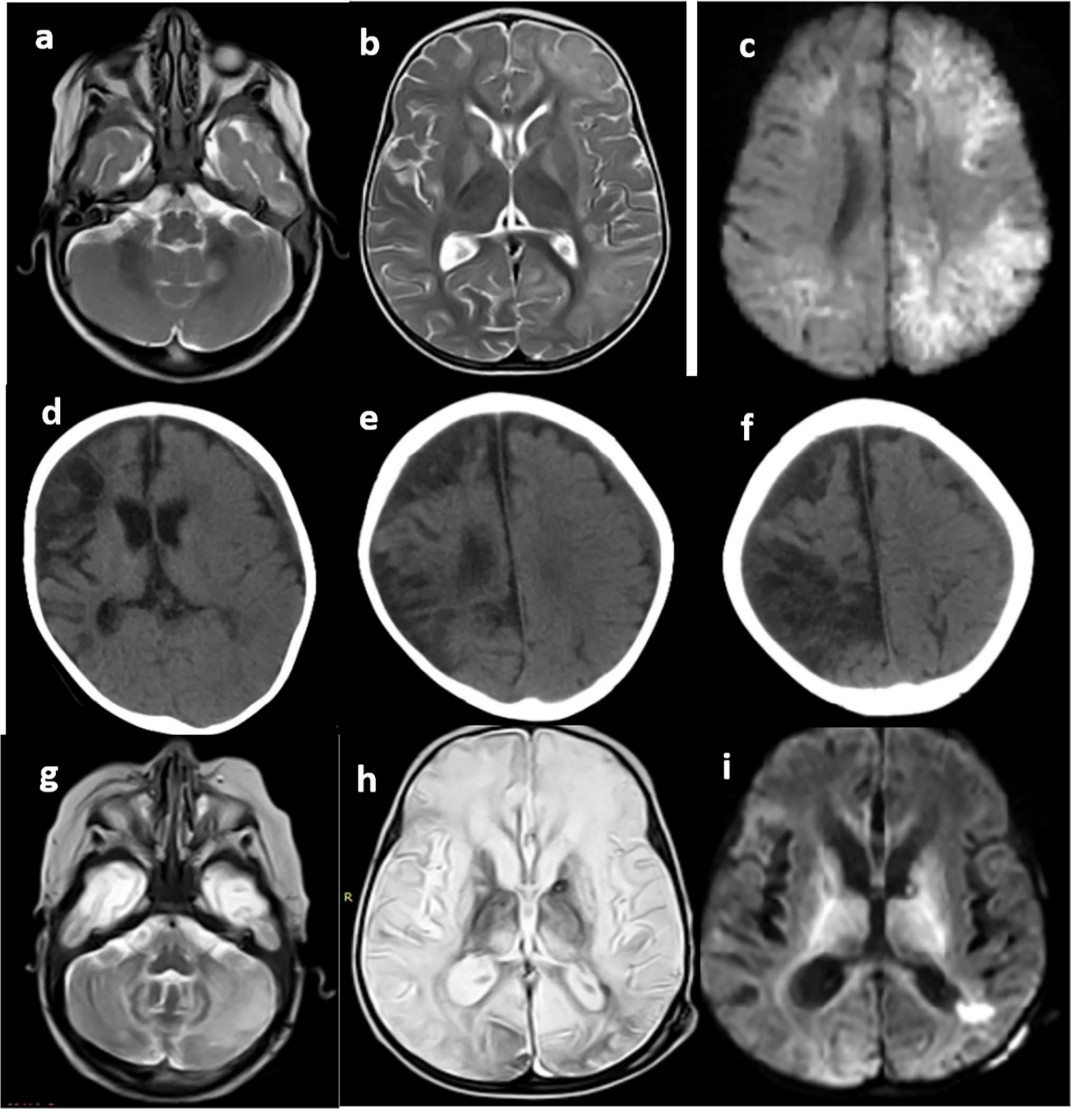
Magnetic resonance imaging manifestations of a patient with *PRF* mutation at initial presentation, follow-up, and central nervous system reactivation. a, b Initial Magnetic resonance imaging axial T2-weighted images showing left cerebral white matter swelling and high T2 signal with patchy high signal involving the left middle cerebellar peduncle. c Axial diffusion-weighted image shows left cerebral patchy high signal of true diffusion restriction. d, e, f Axial computed tomography images at 8-month follow-up show global cerebral atrophic changes with right cerebral gliosis and cystic encephalomalacia. g, h, i Axial T2-weighted image at the cerebellar level (g), T2-weighted image at the supratentorial level (h), and diffusion-weighted (i) images taken at clinical reactivation after 4 months show global white matter gliosis and cerebral atrophy with areas of restricted diffusion
